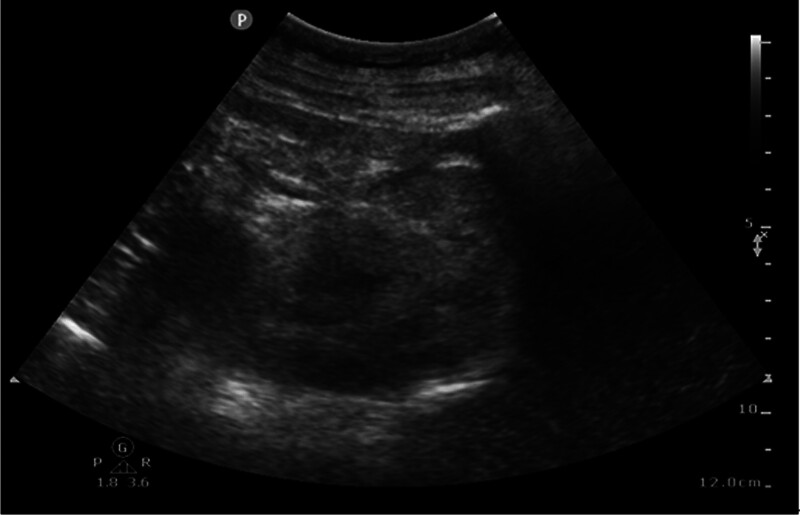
Bedside ultrasound showed an abscess of 7 × 8 cm.
